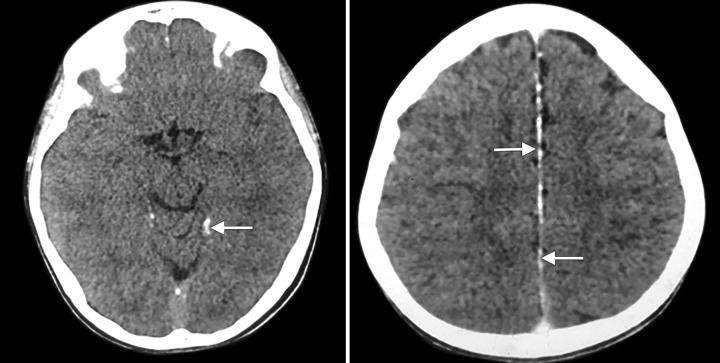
Computed tomography brain showing calcification of falx cerebri and tentorium
